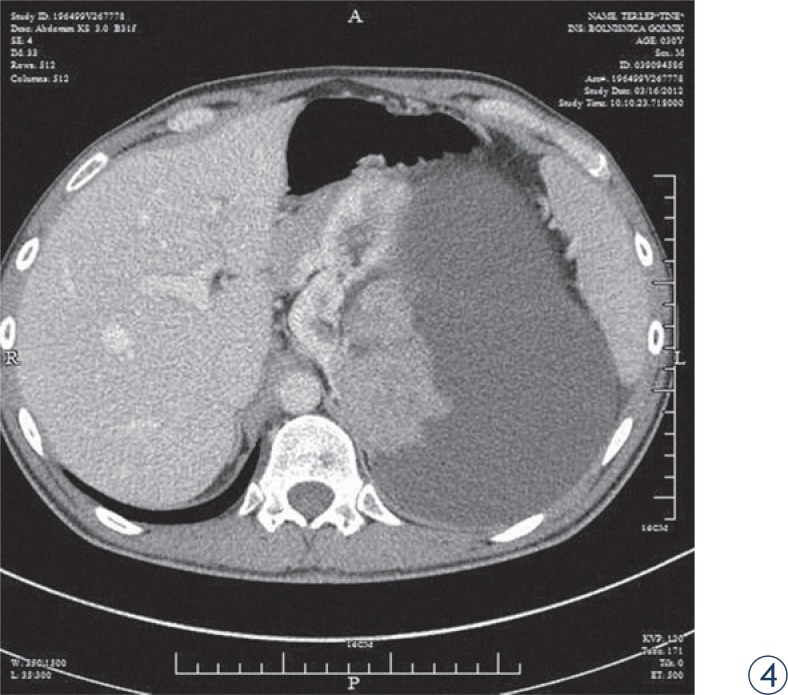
CT scan of the 31 year adult male with cystic mass.
